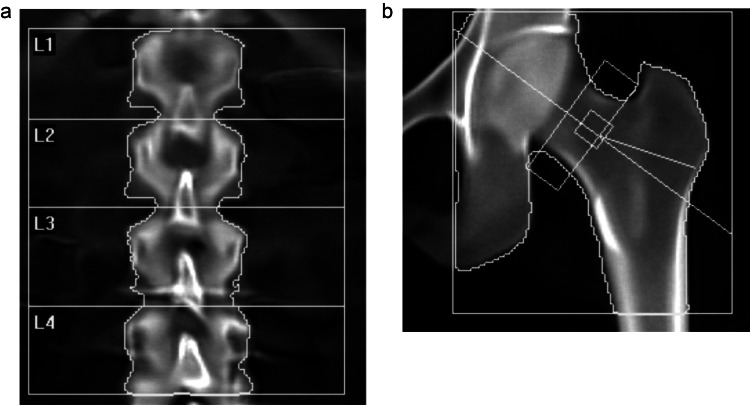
Bone mineral density after conventional intervention from November 2019 to October 2021 Dual-energy X-ray absorptiometry (DXA) scans showed no change in the baseline lumbar spine T-score of -1.0 (a) and only a non-significant improvement in the femoral neck T-score to -1.2 (1.9% improvement, within the error range of the machine) from -1.3 (b) after two years of conventional interventions.
